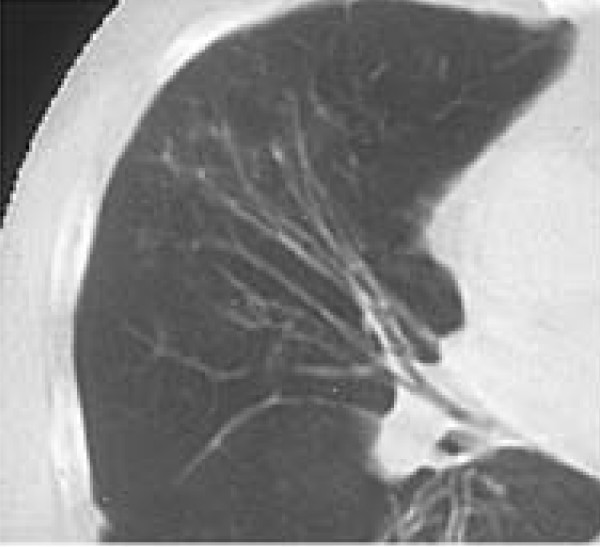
Computed tomography revealed tram-line shadows and cylindrical bronchiectasis in the middle lobe.
